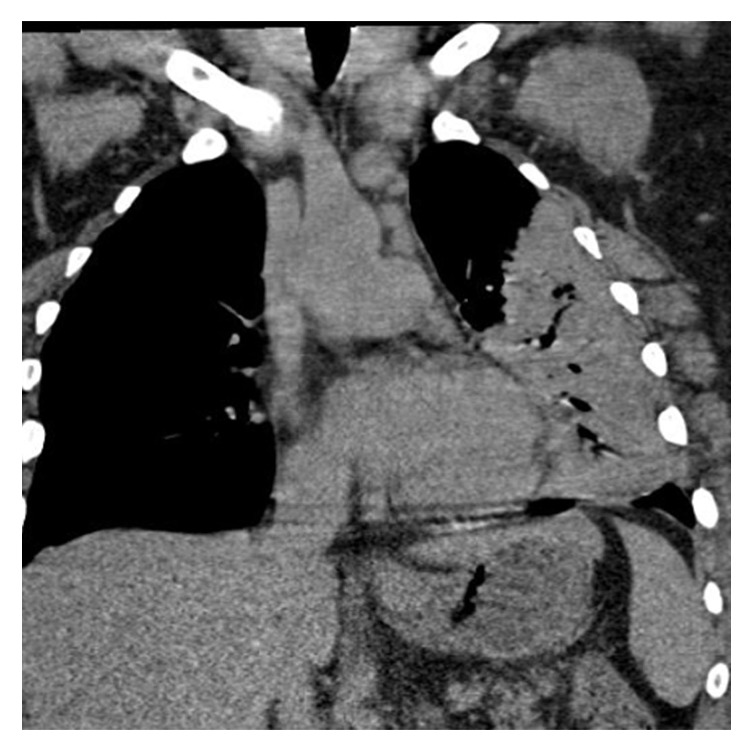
Coronal section chest CT showing consolidation in the left midzone with air bronchograms consistent with lobar pneumonia, without pleural effusion.
